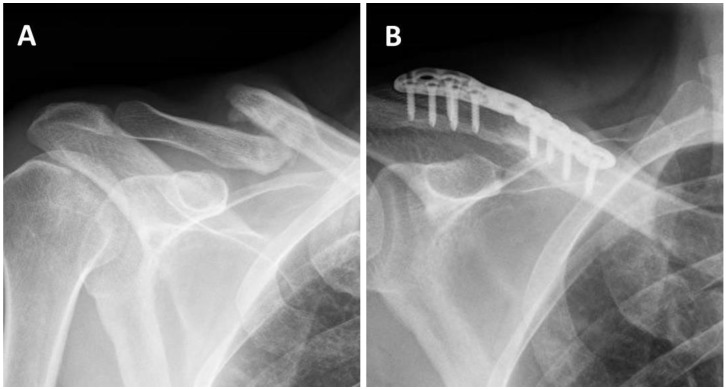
A 47 year old patient who suffered from symptomatic right–middle clavicle nonunion after conservative treatment: preoperative X-ray (A) at 5 months after the injury, and (B) at 1 year following ORIF with supplementation of BMAC. ORIF; open reduction and internal fixation BMAC; bone marrow aspirate concentrate.
